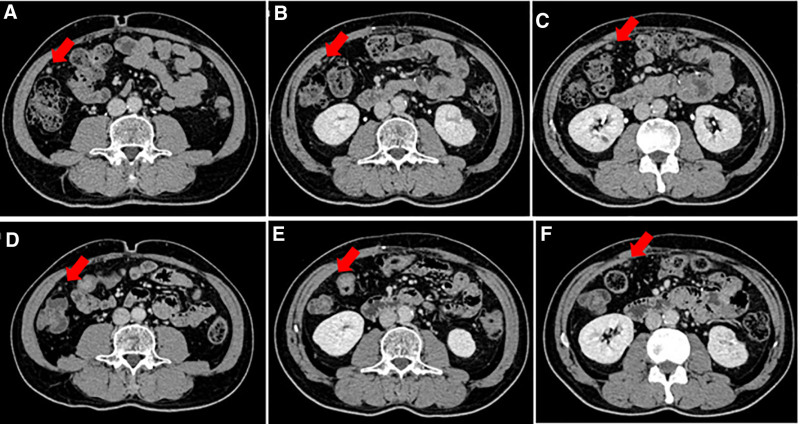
CT showed partial response of the disease, before the use of T-DXd (A–C) and after 2 cycles of T-DXd treatment (D–F); CT = computed tomography; T-DXd = Trastuzumab deruxtecan.
